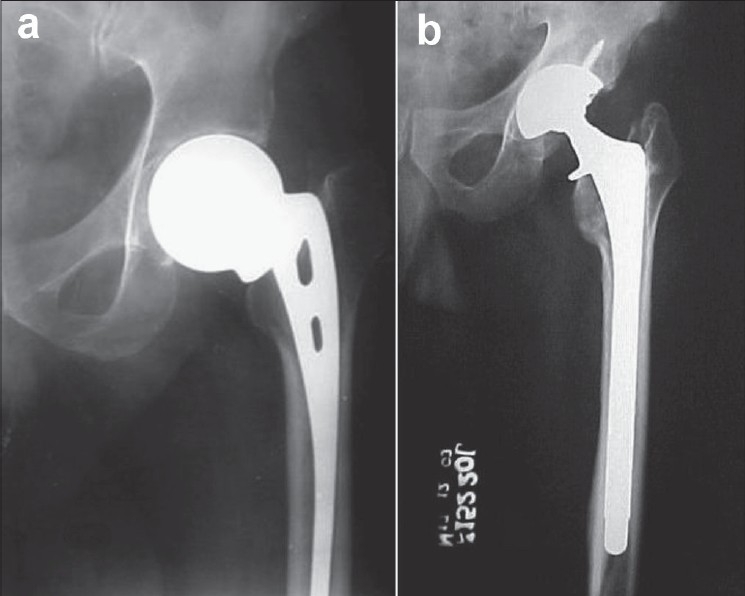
(a) Preoperative and, (b) 48 months follow-up X-rays of conversion of a loose Austin Moore prosthesis to a cementless total hip arthroplasty (THA) with long femoral stem. A non-progressive radiolucent line less than 2 mm in thickness was noted in Zone 7
